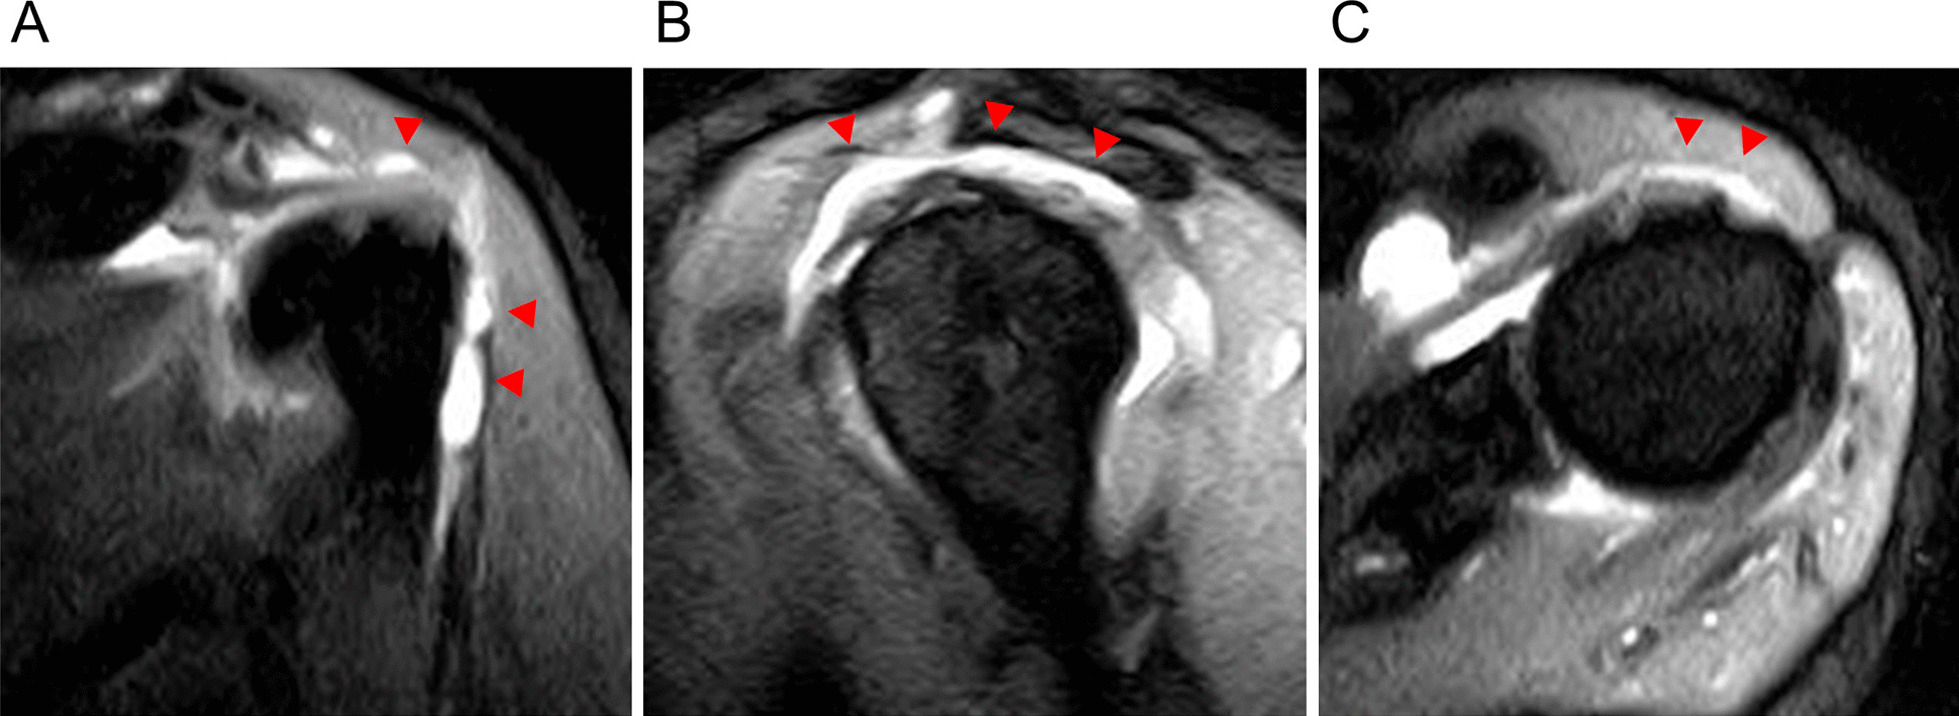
Short TI inversion recovery of magnetic resonance imaging of the left shoulder (initial visit). A Coronal view, B sagittal view. C axial view. Images 5 months after receiving the COVID-19 vaccine demonstrate effusion in the subacromial–subdeltoid bursa suggesting bursitis (arrowheads)
